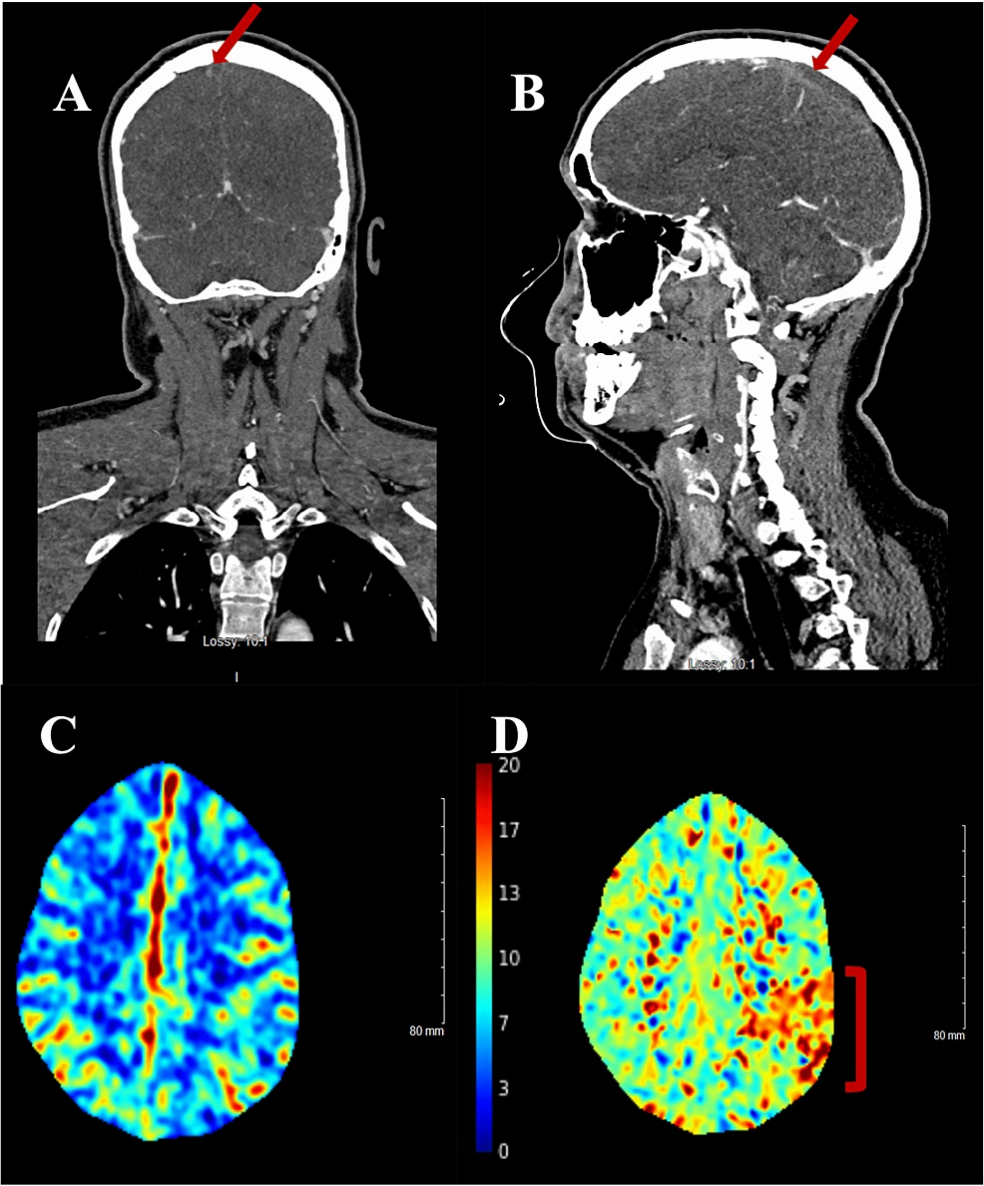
CT Angiogram and Perfusion Scans of the Head (A) Coronal and (B) Sagittal CTA demonstrating the absence of blood flow in the superior sagittal sinus. (C) Normal symmetric bilateral CBV on CT perfusion. (D) MTT sequence of the CT perfusion scan showing increased mean transit time in the left parietal lobe. CT: computed tomography; CTA: computed tomography angiogram; CBV: cerebral blood volume; MTT: mean transit time
